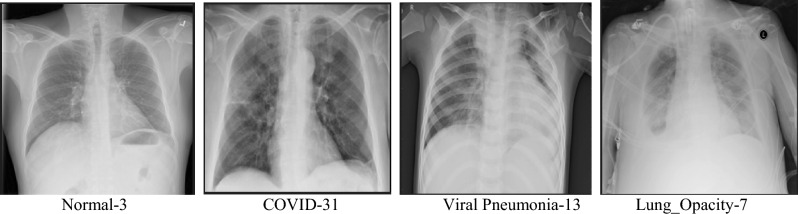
Samples from COVID-19 radiography chest database representing different classes.
